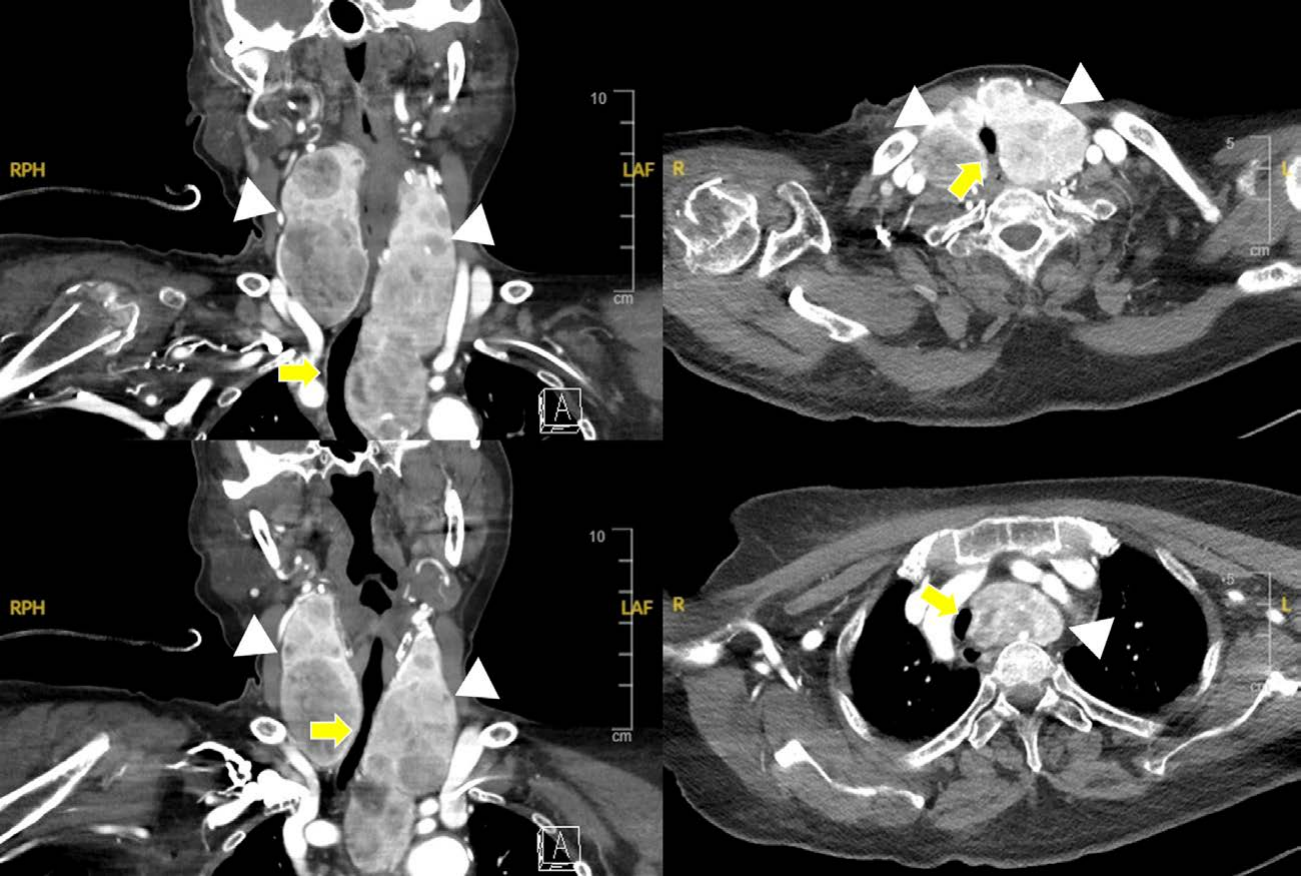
Neck computed tomography. A huge multinodular goiter (white arrow head) extends into the retrosternal region and compresses the trachea (yellow arrow), causing it to be deviated to the right.
